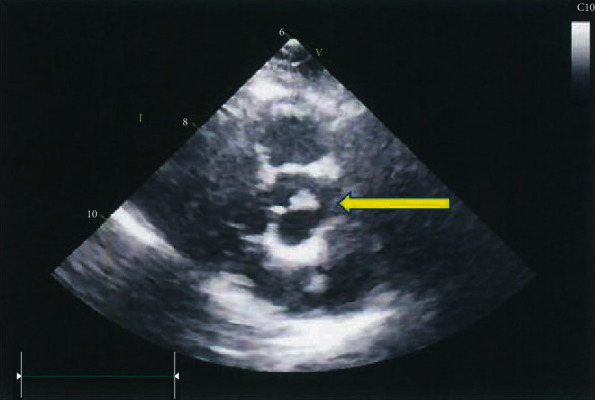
Transthoracic echocardiography: parasternal short axis shows a round mass, 9 mm in diameter (yellow arrow), on the aortic side of the right coronary cusp of the aortic valve.
